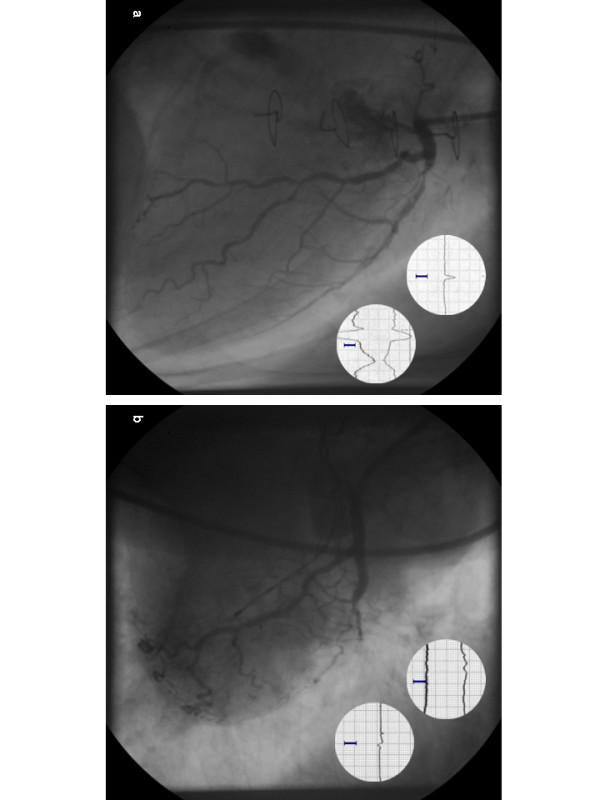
(a) Chronic total occlusion of the right coronary artery with vital and ischemic myocardium of the posterior wall (scintigraphy, data not shown). b) Retrograde filling of the right coronary artery via injection into the left coronary artery; note simultaneous placement of the catheter in the right coronary and left coronary artery respectively in (a) and (b). (c) PCI wire in the distal right coronary artery. (d) Verification of intraluminal seating of the PCI wire in the right posterior descending artery with retrograde filling via the catheter in the left coronary artery. (e) Extensive dissection of the right coronary artery after balloon angioplasty. (f) Complete and stable revascularization after stent implantation.
